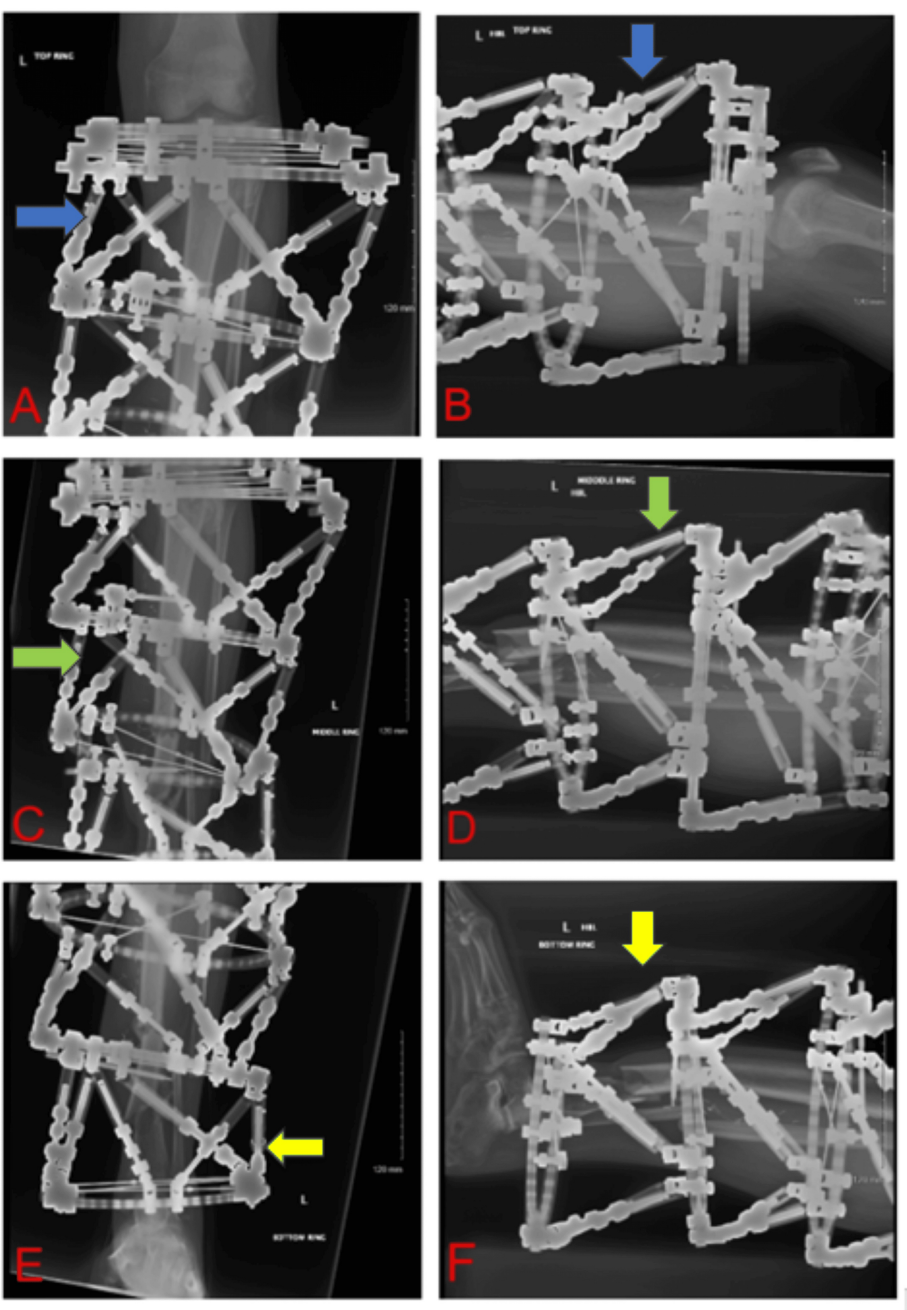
Postoperative radiographs showing a triple-stacked Taylor Spatial Frame (TSF) applied, with alignment correction planned using TSF software Following the initial postoperative radiographs, alignment correction is initiated using the TSF software. For each segment mounted with a hexapod that contains a primary fracture line, radiographs must be obtained in two orthogonal planes: anteroposterior and lateral. In each instance, the central X-ray beam must be oriented perpendicular to the fracture plane. Consequently, a total of four radiographs is required for double-stacked constructs, and six for triple-stacked configurations [7]. All images presented are radiographs of the affected left tibia treated with a triple-stacked hexapod external fixator. A: Anteroposterior radiograph focused on the proximal hexapod segment of the left tibia (blue arrow). B: Lateral radiograph of the proximal hexapod segment of the left tibia (blue arrow). C: Anteroposterior radiograph centred on the middle hexapod segment, depicting the tibial shaft fracture (green arrow). D: Lateral radiograph focusing on the middle TSF rings and struts and their fixation to the tibial shaft segments (green arrow). E: Anteroposterior radiograph of the distal hexapod segment at the distal tibia and ankle level (yellow arrow). F: Lateral radiograph of the distal hexapod segment at the distal tibia (yellow arrow). ^These radiographic images were obtained in our hospital during the course of the patient’s treatment. Informed consent was obtained for their publication. The patient is under our care in the limb reconstruction unit, and the surgical procedures were performed by two of the authors as part of the overall treatment plans.^
